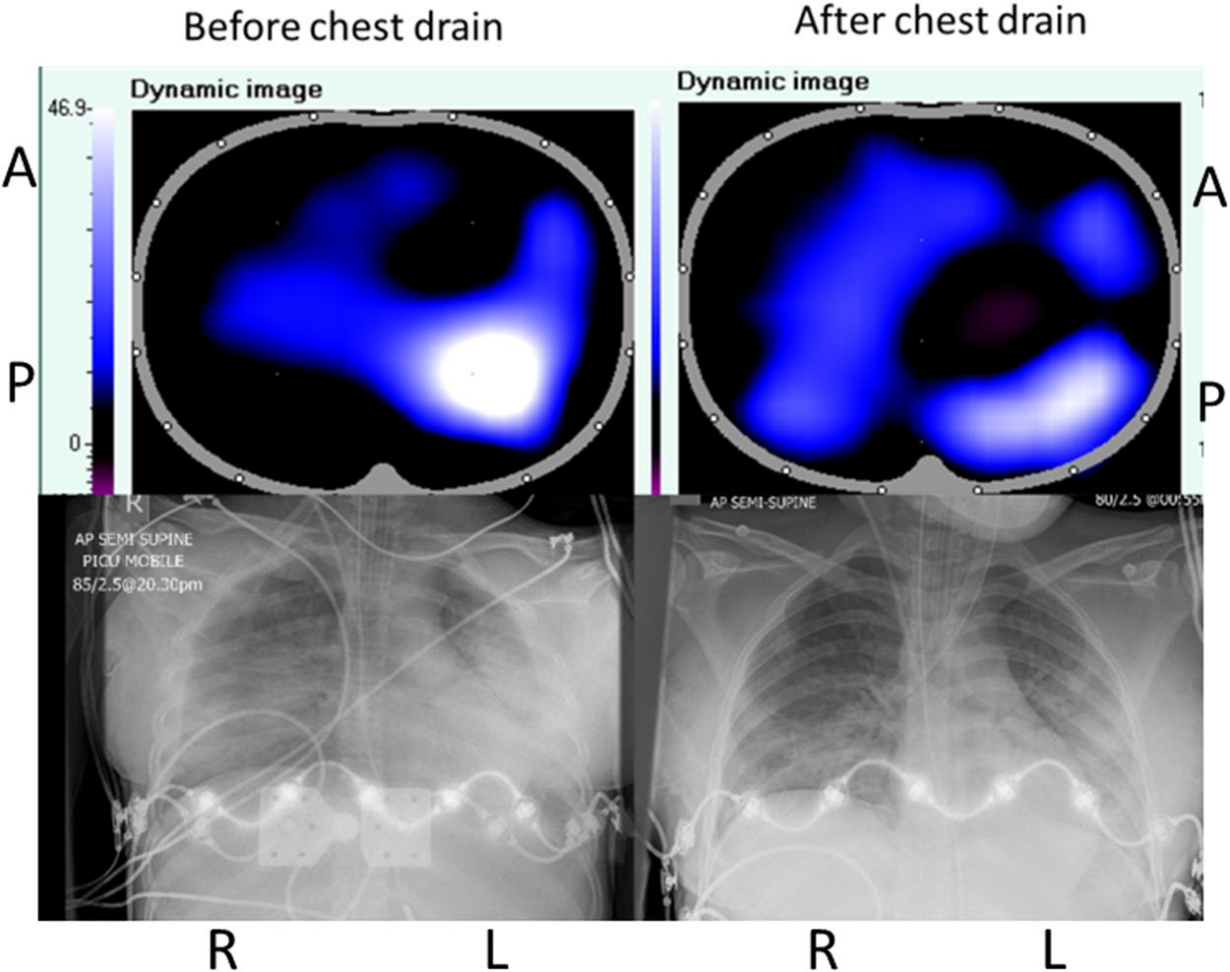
Pre and post chest drain live impedance images, with concurrent radiographs. Live tidal EIT images show the impedance change in each area, with brighter (whiter) pixels denoting a greater impedance change, equivalent to increased ventilation, in that area.
